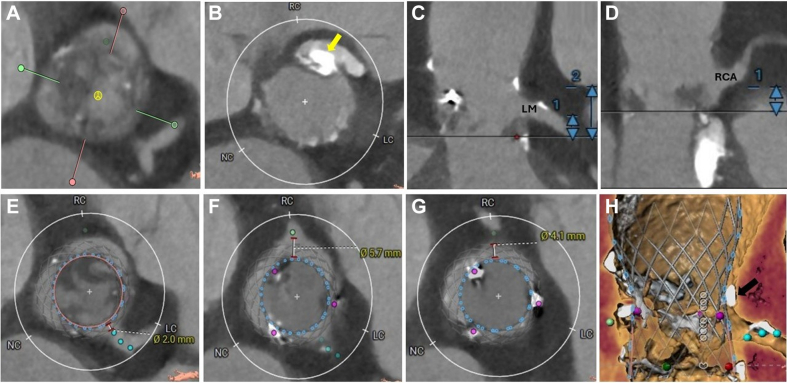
Case 1: Preprocedural Computed Tomography Angiography and Virtual Modeling of the Transcatheter Heart Valve (A to D) Computed tomography angiography showing thickened Mosaic valve leaflets (A), caseous calcification below the right coronary cusp (yellow arrow in B), and relatively low coronary heights from the aortic valve annulus: 6.9 mm for the LM (C) and 7.4 mm for the RCA (D). (E to H) Modeling of a 26-mm Evolut FX+ transcatheter heart valve showed a very short VTC distance of 2 mm (E) for the LM (black arrow in H) and 5.7 mm for the RCA (F), with a VTA distance of 4.1 mm (G). LC = left coronary cusp; LM = left main coronary artery; NC = noncoronary cusp; RC = right coronary cusp; RCA = right coronary artery; VTA = valve to aorta; VTC = valve to coronary.
